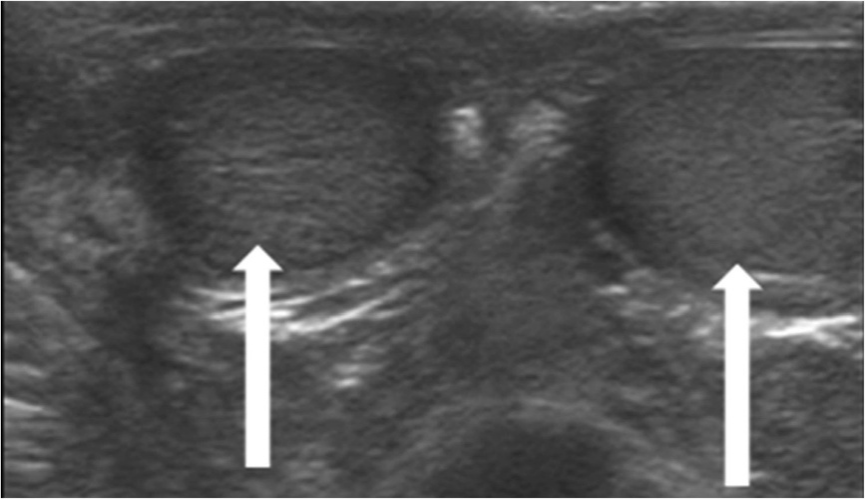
Grayscale ultrasound of patient 2. Image shows a normal testis on each side of the scrotum (white arrows).
